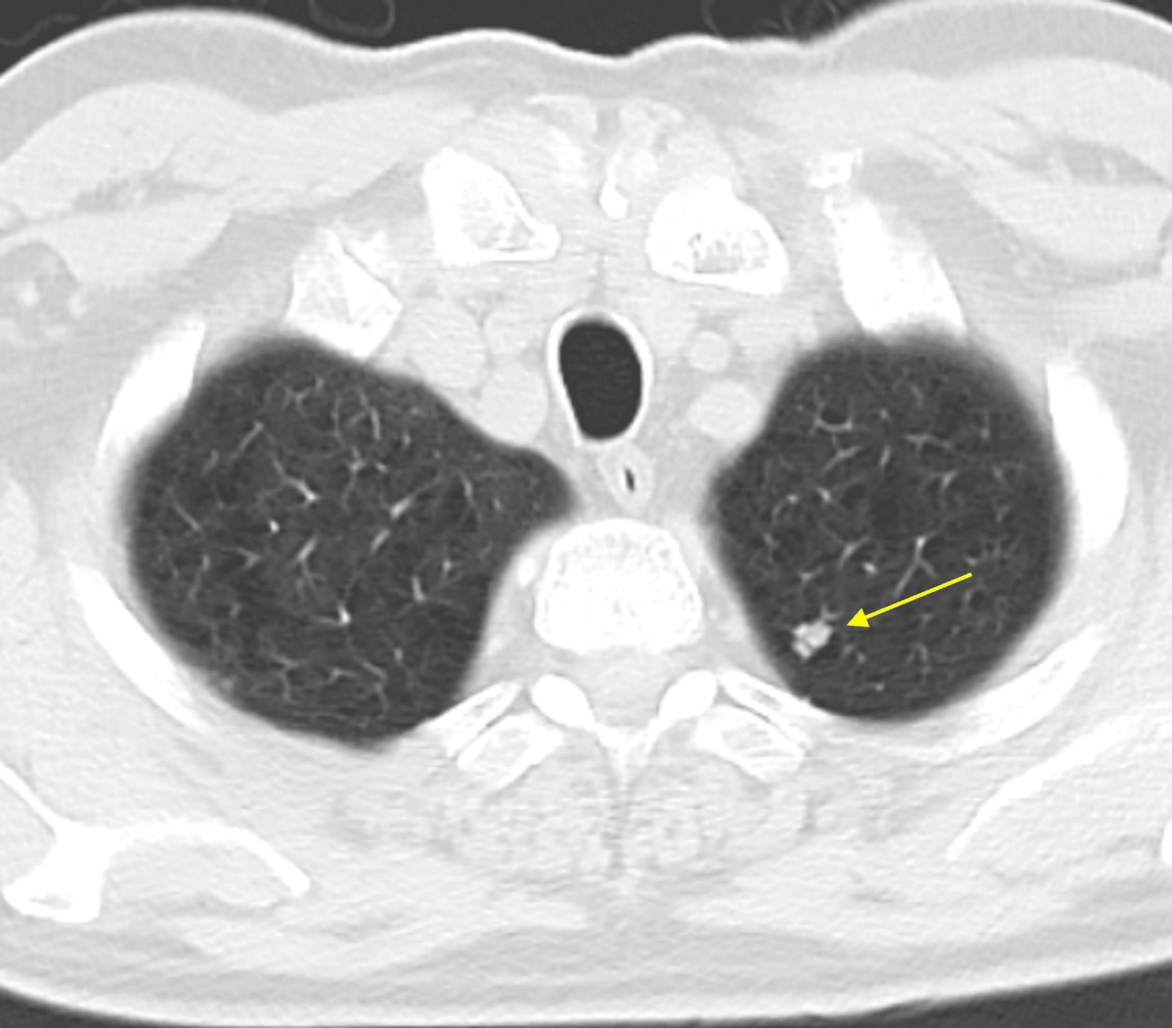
Chest CT scan in March 2024 showing a 8 x 6 mm nodule in the upper lobe of the left lung CT: computed tomography
